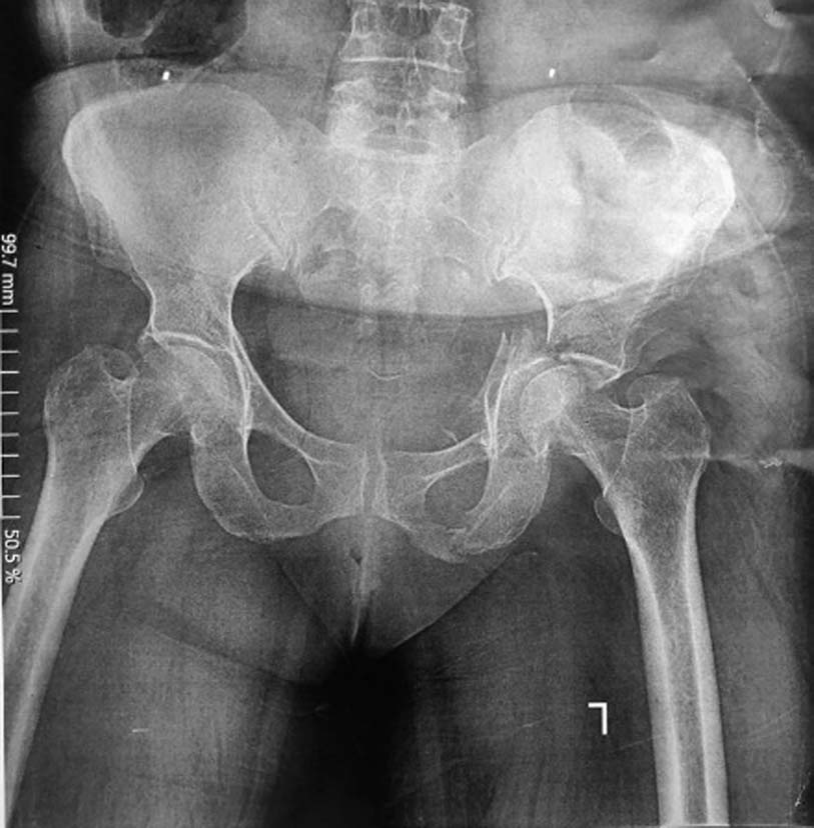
Plain pelvic radiograph before the fixation surgery (anteroposterior view).
